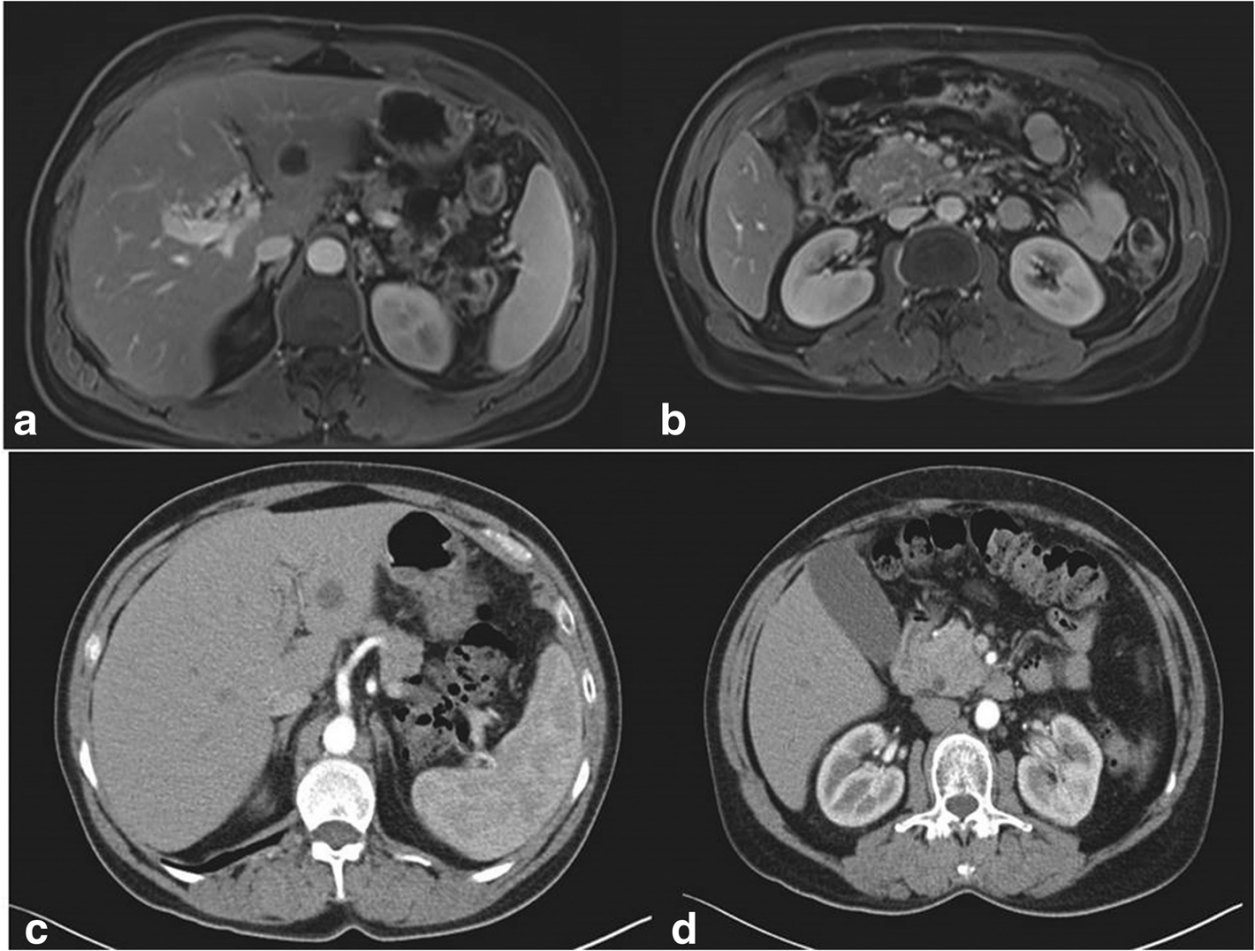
a MRI showing suspicious lesion in segment III of the liver prior to chemotherapy. b MRI showing pancreatic mass along with thrombosis of SMV prior to chemotherapy. c CT showing downsizing and minimal response of the segment III liver lesion after chemotherapy. d CT showing downsizing and minimal response of pancreatic mass after chemotherapy
